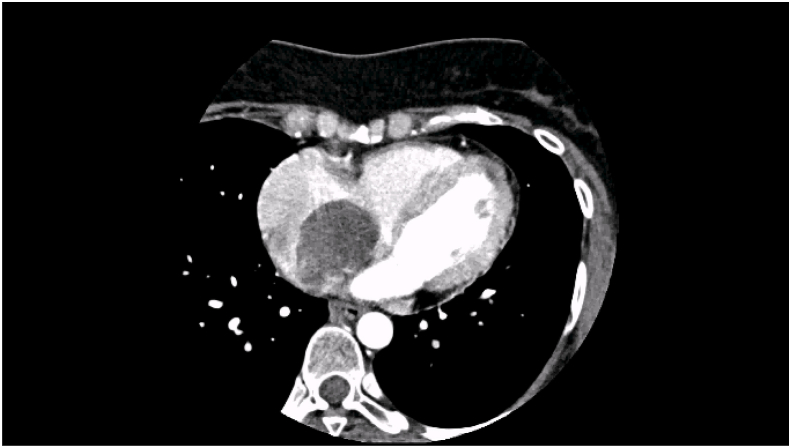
CT Angio showing 46 × 40 × 40 sized hypo density seen in right atrium minimal extension into IVC atrial junction.
